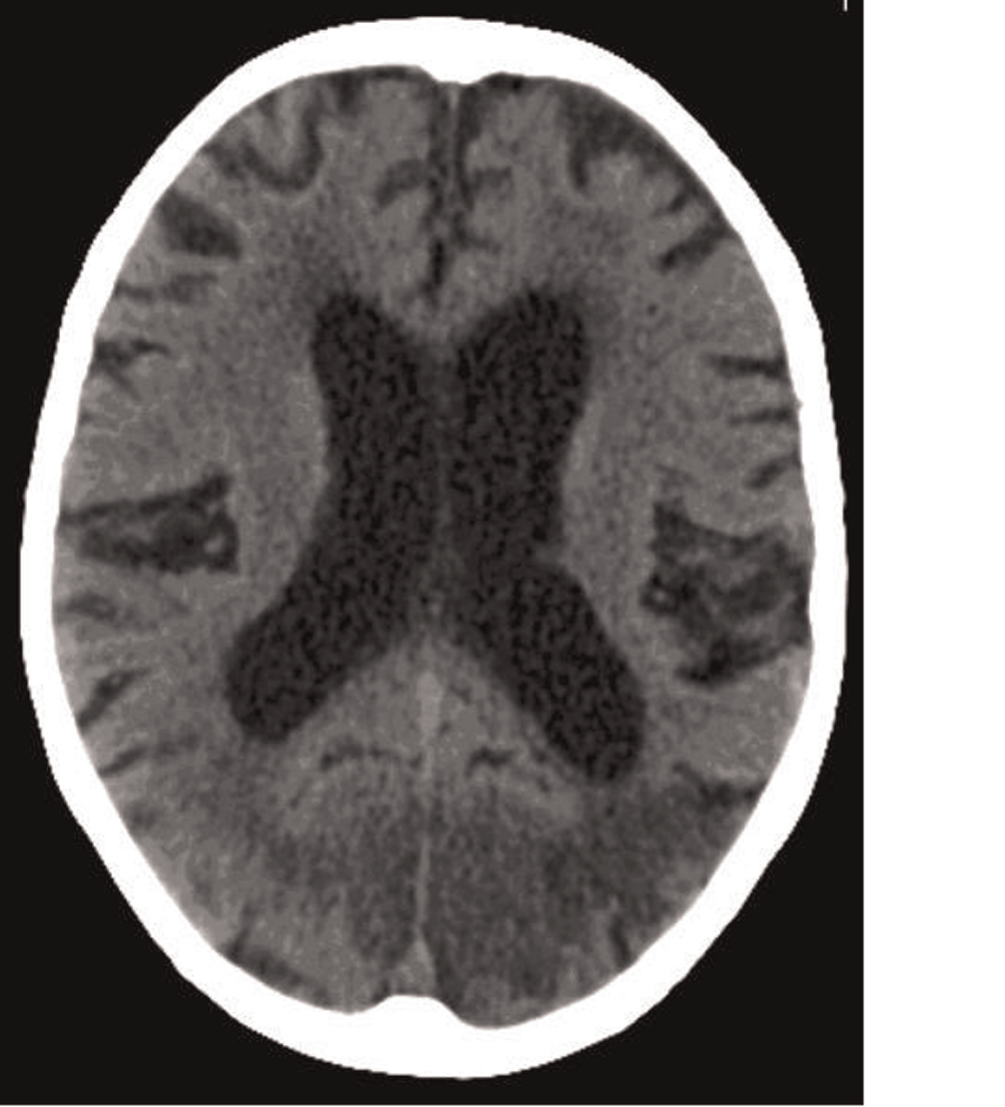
A computed tomography scan of the patient's brain at initial presentation, demonstrating acute infarction in the right occipital and left occipito-parietal lobes.
